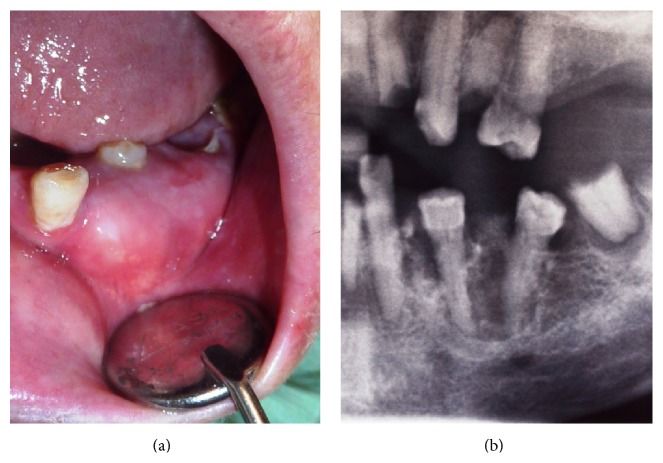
(a) Intraoral view of a left mandible enlargement with displacement and submersion of involved teeth. (b) Panoramic radiographic image (partial view) showing a multilocular radiolucent lesion with calcified foci.
